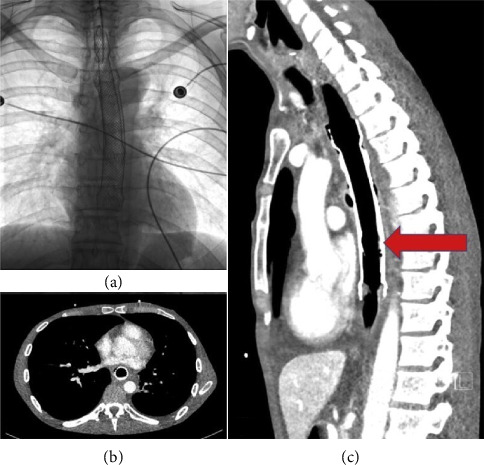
(a) Fluoroscopy showing the radiologic appearance of a stent-in-stent at the time of esophageal stent implantation. (b and c) Computed tomography, transverse, and sagittal sections showing partial compression of the posterior left atrial wall by an esophageal stent. Note the termination of the inner stent at left atrium level (red arrow) (adapted from Mazzella et al.) [20].
